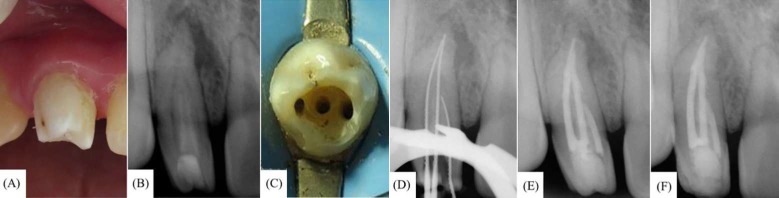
A)* Crown of maxillary right lateral incisor exhibits a cone-shaped morphology; *B)* Pre-operative radiograph showing Oehlers type II dens invaginatus (DI); *C)* Access cavity depicting three canal orifice entrances; *D)* Working length determination; *E)* Post-operative radiograph; *F)* Follow-up at six months
